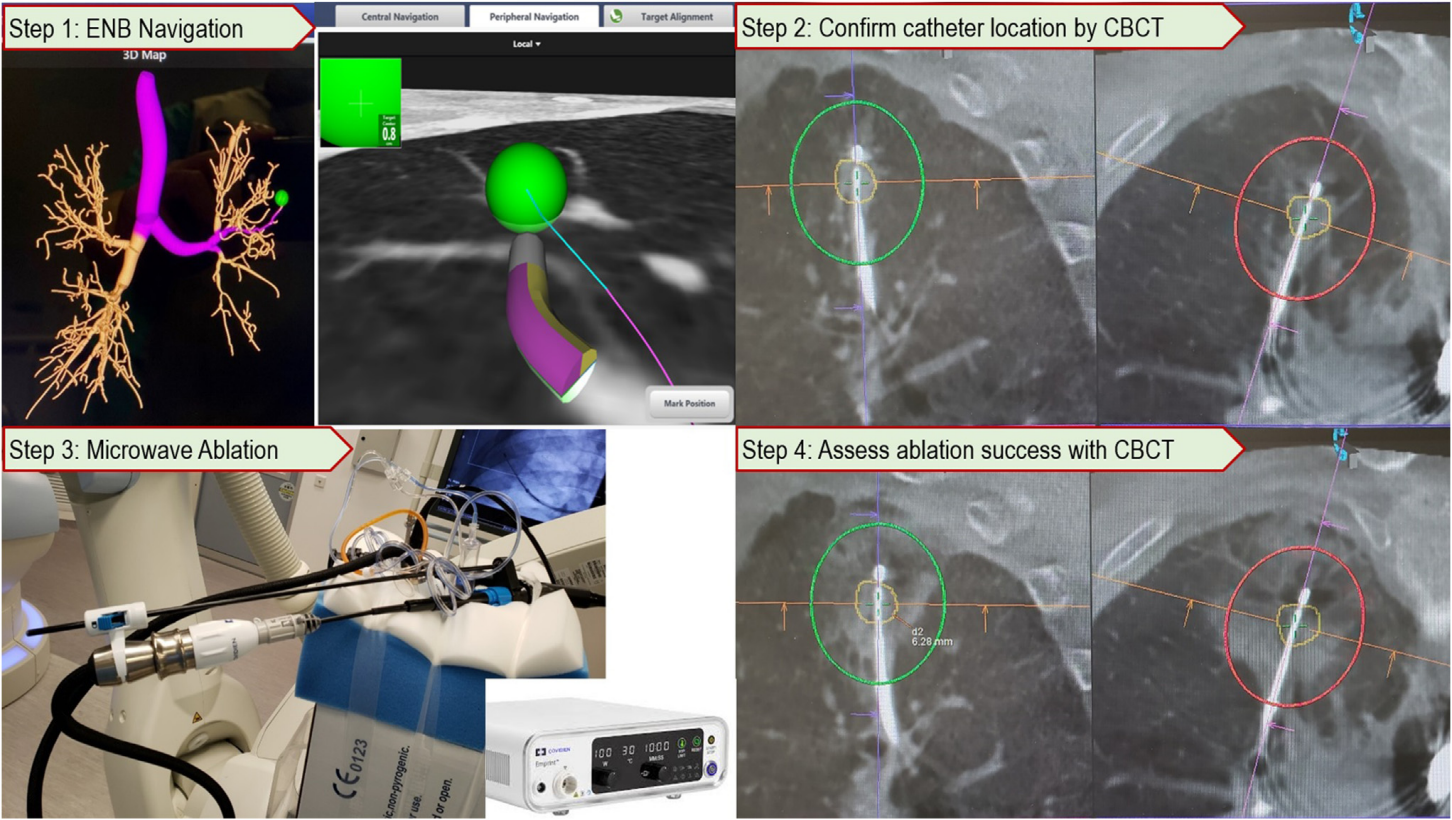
Key procedural steps of transbronchial MWA using ENB are shown. The first step consists of navigation toward the lesion (*green ball*) using ENB guidance. The second step includes confirmation of locatable guide in close proximity to lesion, exchanging it to ablation catheter with or without transbronchial access tools, and final unsheathing of the ablation catheter followed by a preablation CBCT. In this case, the lesion is marked by orange outline, punctured by ablation catheter, and the green and red ovals represent the predicted ablation zones. The third step includes MWA, the level of energy used depending on intended ablation size. The last step includes a postablation CBCT showing the actual ablation zone represented by ground-glass opacities covering the original lesion. *ENB*, Electromagnetic navigation bronchoscopy; *CBCT*, cone beam computed tomography.
